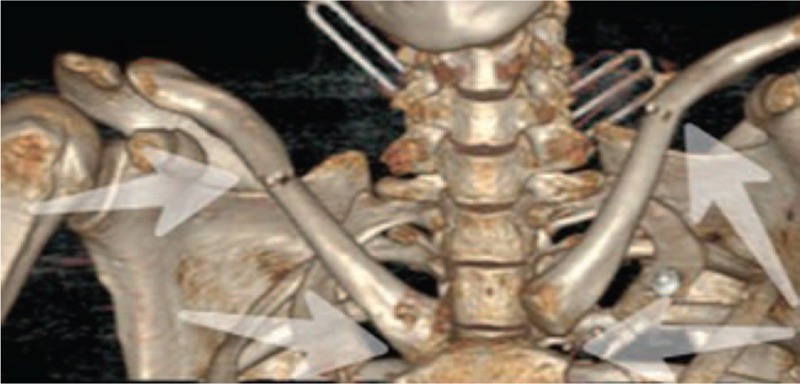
A computed tomography (CT) scan with three-dimensional reconstructions of sternoclavicular joint (SCJ) showed potentially life-threatening posterior dislocation of right SCJ and anterior dislocation on the left SCJ combined with bilateral clavicular fracture. CT = computed tomography, SCJ = sternoclavicular joint.
